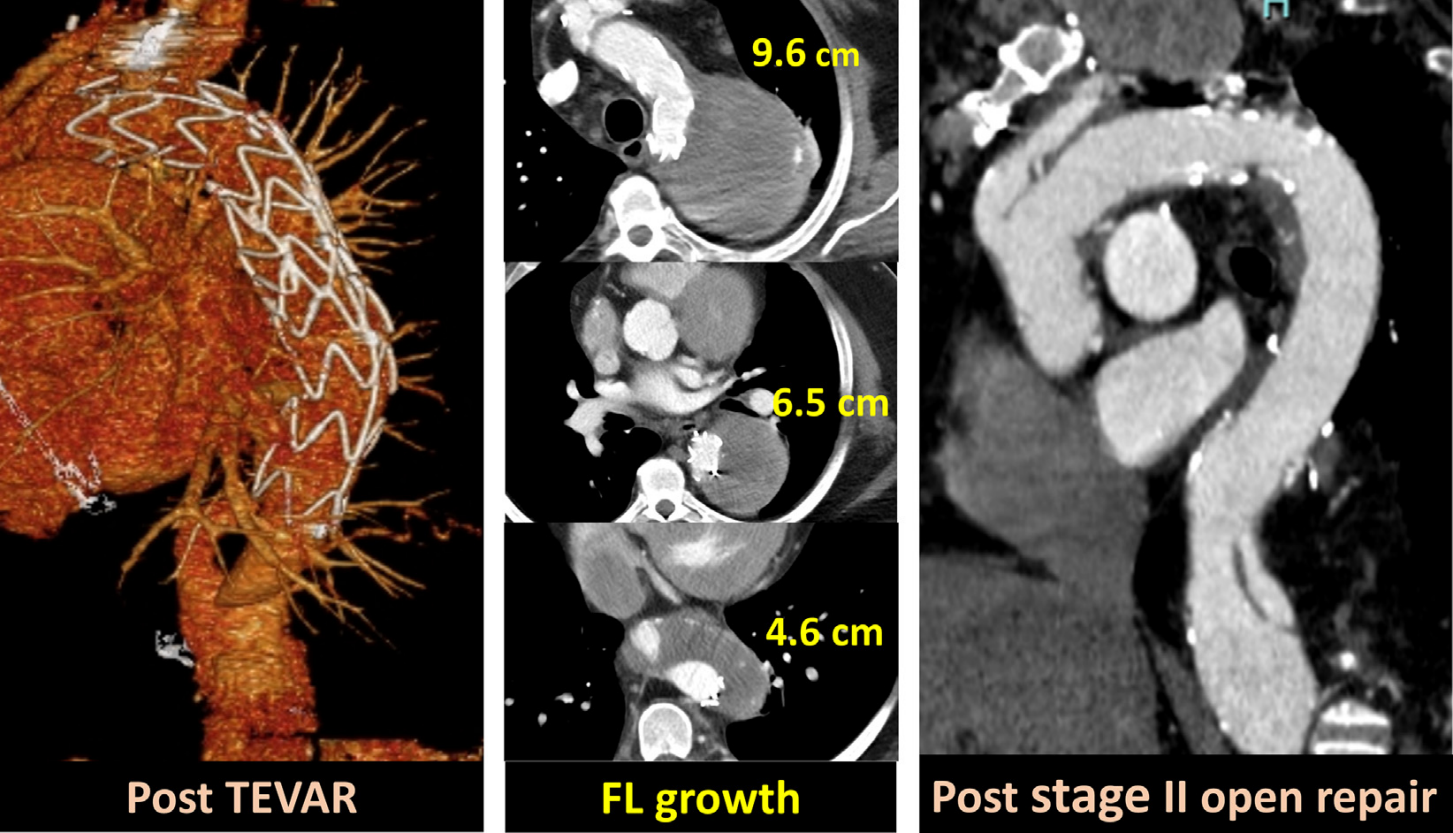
Computed tomography of a patient who underwent TEVAR. Open descending aortic repair was required due to expansion of downstream aorta 5 years after TEVAR. *TEVAR*, Thoracic endovascular aneurysm repair.
